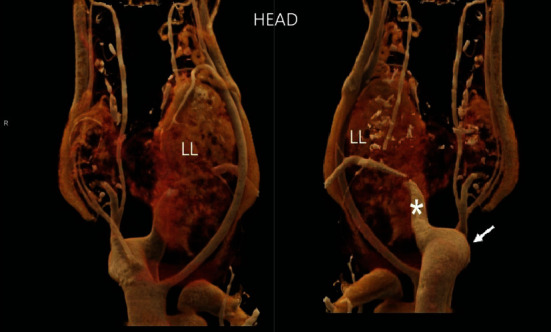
CT scan three-dimensional reconstruction. Frontal and posterior views of the cervical region, showing the volumetric increase in the thyroid, pronounced on the left lobe (LL), and evidencing the presence of the aortic arch on the right side (arrow), with left subclavian artery with retroesophageal path (asterisk).
